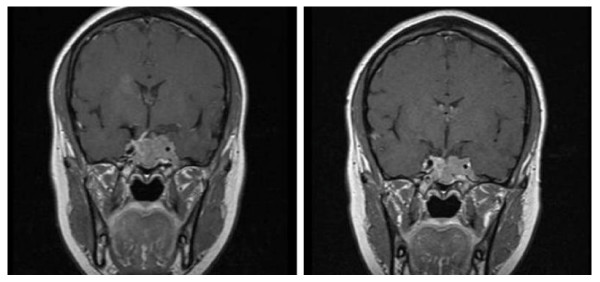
(A) Magnetic resonance imaging scan showing the tumor size before lanreotide treatment. (B) Magnetic resonance imaging scan showing a reduction in tumor size after 10 months of lanreotide treatment in Case 1.
